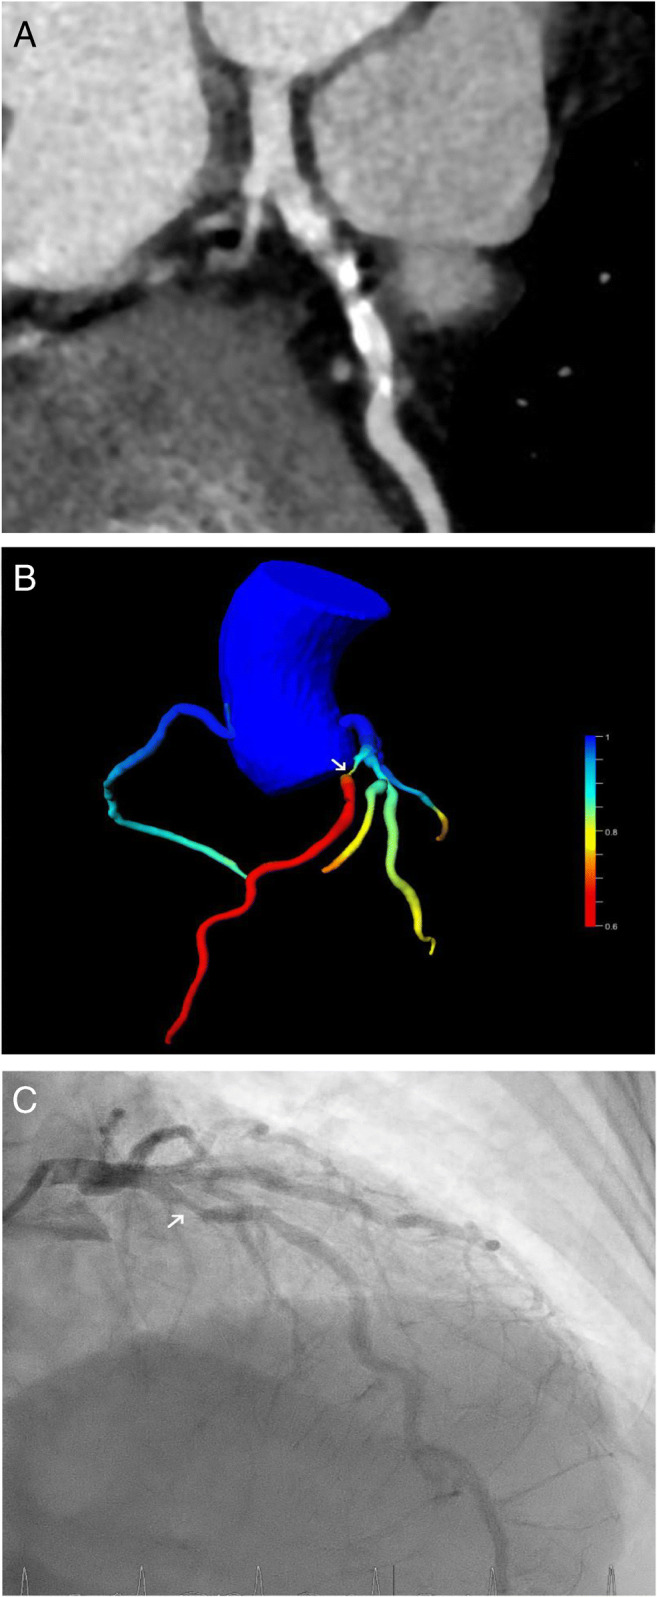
A 61-year-old female patient undergoing evaluation for liver transplantation. A Curved multiplanar reconstruction of the CCTA: A significant stenosis of the LAD was suspected (vessel lumen pre-stenosis 11.6 mm^2^, in-stenosis 4.2 mm^2^, post-stenosis 8.6 mm^2^); B Calculation of CT-FFR revealed hemodynamically significant stenosis (→, CT-FFR 0.43); C This finding was confirmed in ICA (→, iFR 0.7)
